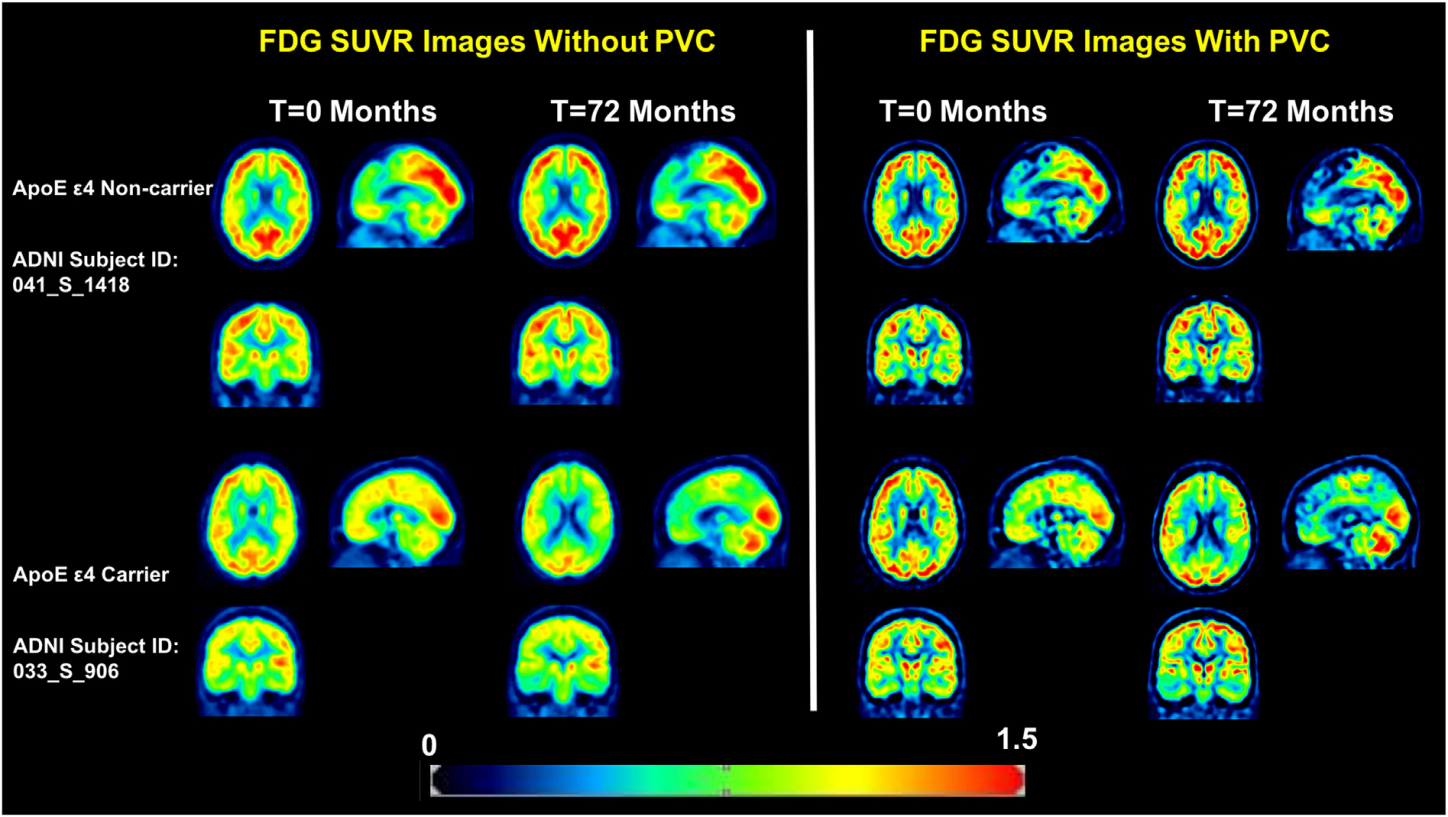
Typical single-subject FDG-PET Image with PVC and non-PVC. Representative cross-sectional slices from selected ApoE ε4 carriers (subject ID: 041_S_1418) and non-carriers (subject ID: 033_S_0906) with and without PVC are displayed to demonstrate the effect of PVC at the single-subject level.
